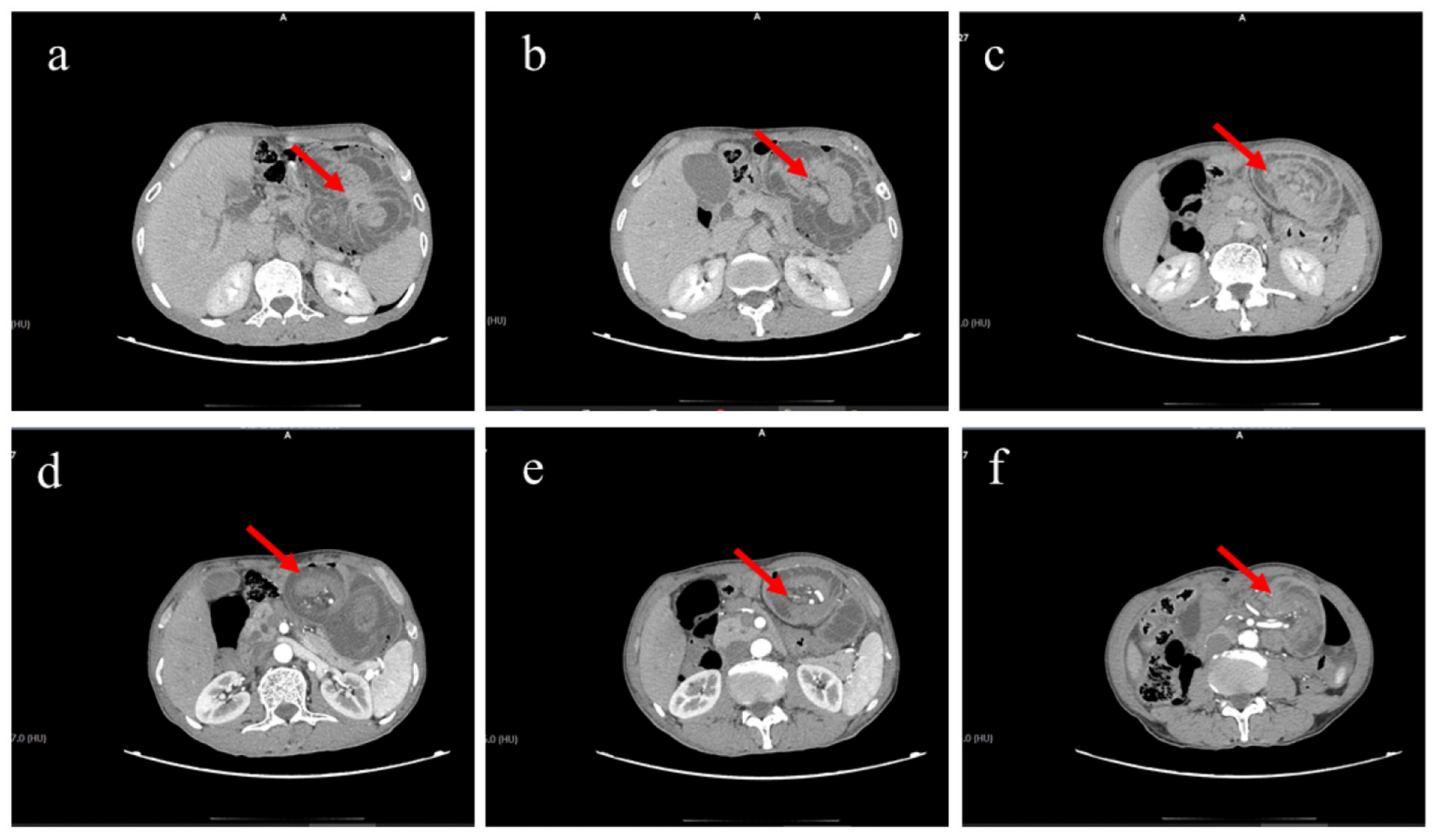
Emergency abdominal CT findings. (a–c) Non-contrast CT images showing thickened small bowel wall in the left upper quadrant (arrows), with partial herniation of small bowel into the gastric lumen and intussusception. (d–f) Contrast-enhanced CT images revealing small bowel volvulus in the left upper quadrant and gastrojejunal intussusception (arrows).
